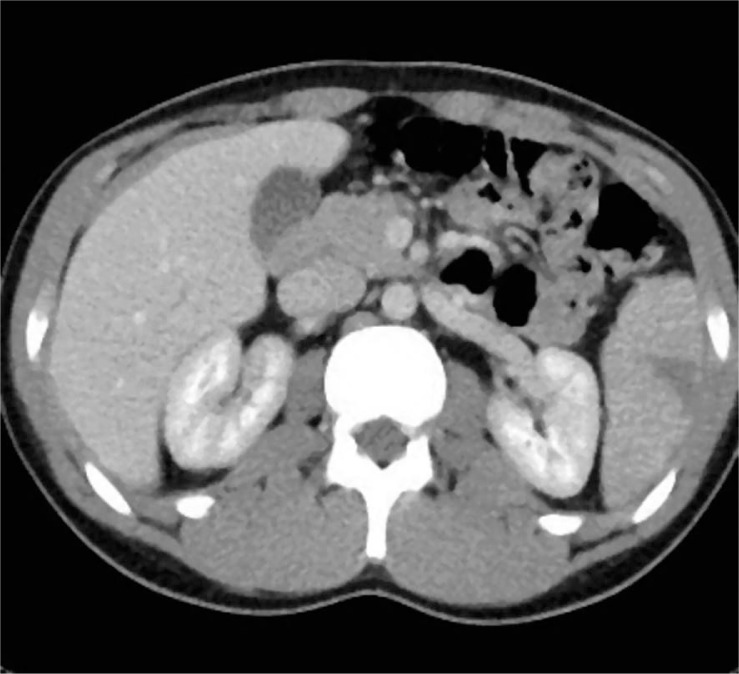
Transverse CT scan, obtained one month after the embolization shows small area of parenchymal infarct.
